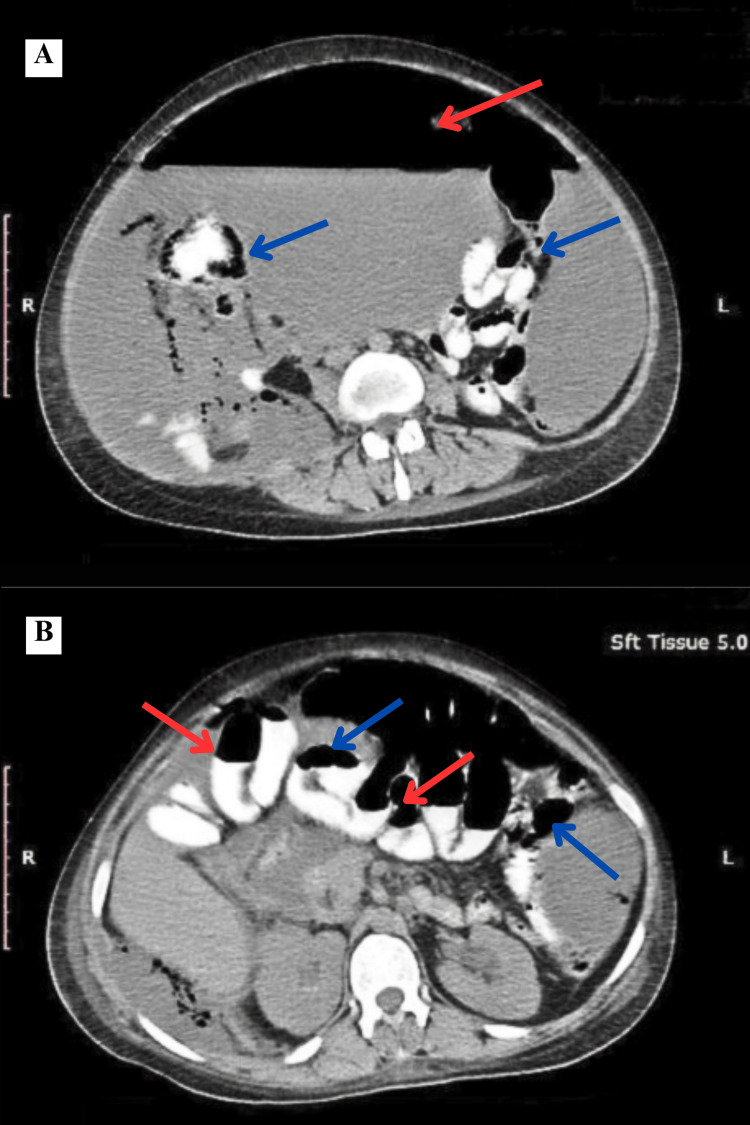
CT of the abdomen and pelvis axial view (oral and IV contrast). The image shows evidence of massive abdominopelvic ascites with large air-fluid levels (red arrows) and multiple air lucencies (blue arrows) representing pneumoperitoneum. IV: intravenous
